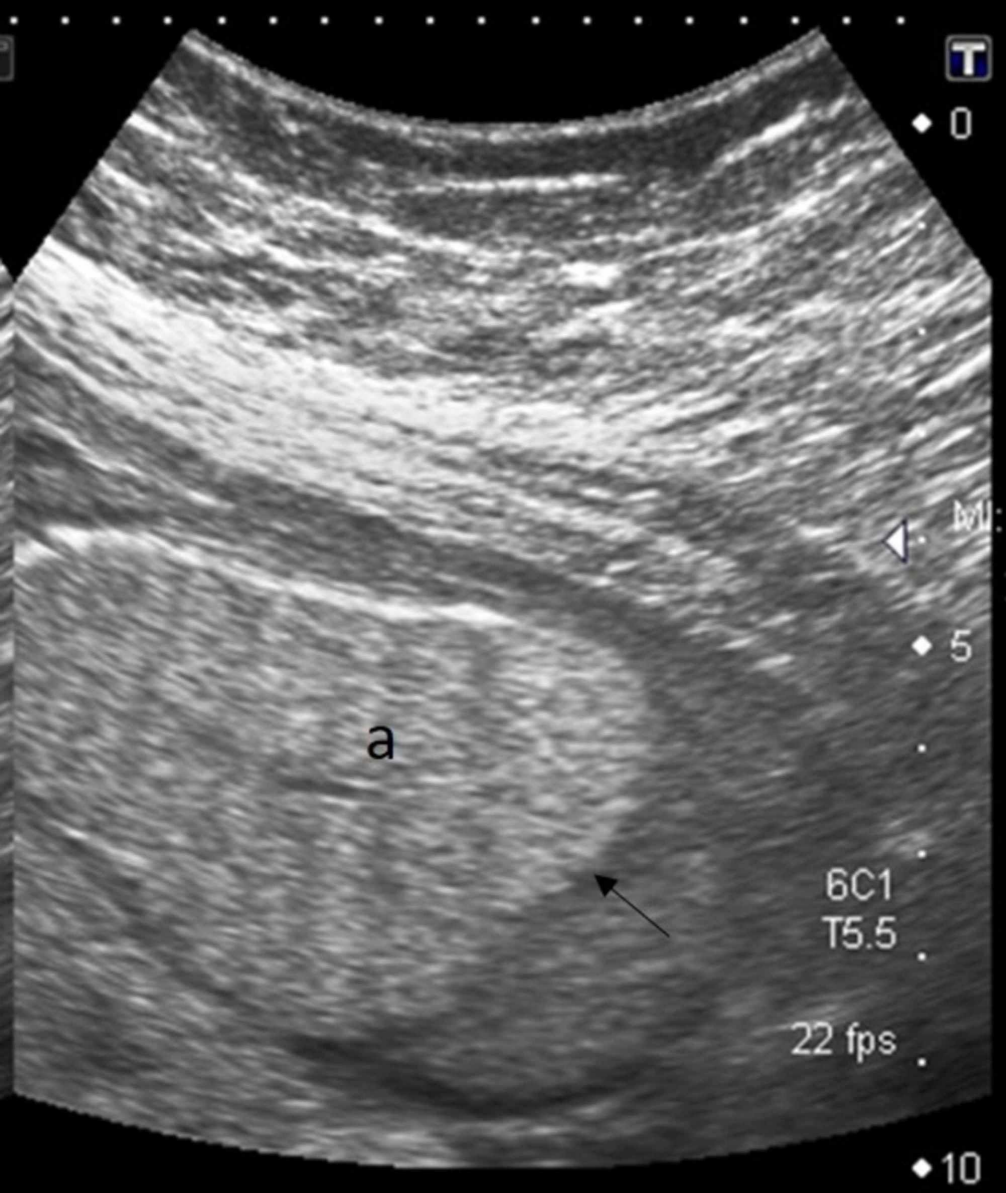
Parasagittal ultrasound image shows inverted diaphragm (black arrow) due to enlarged echogenic lung (a)
